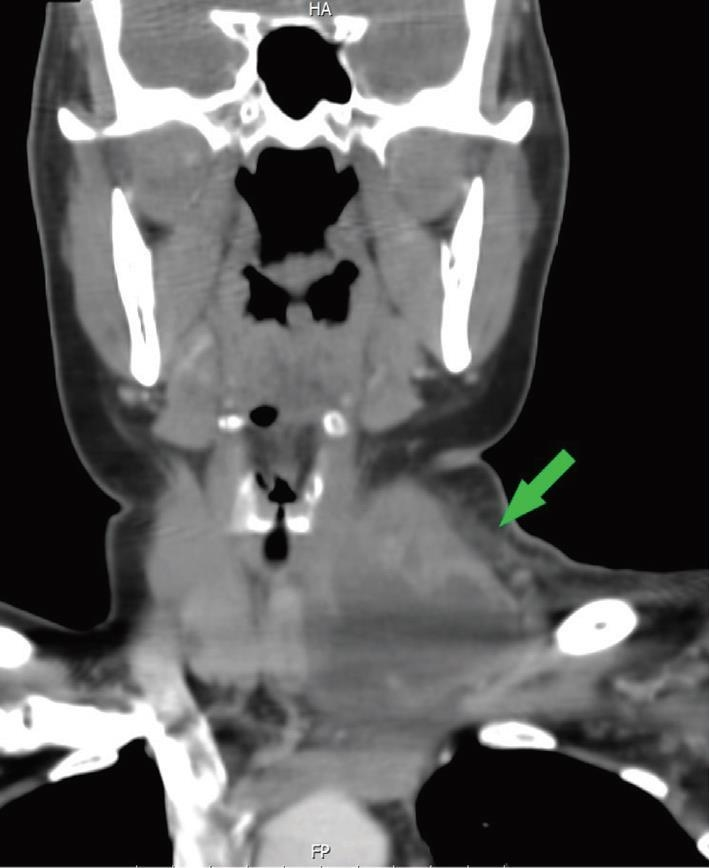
CT scan shows a coronal view of the abscess.
